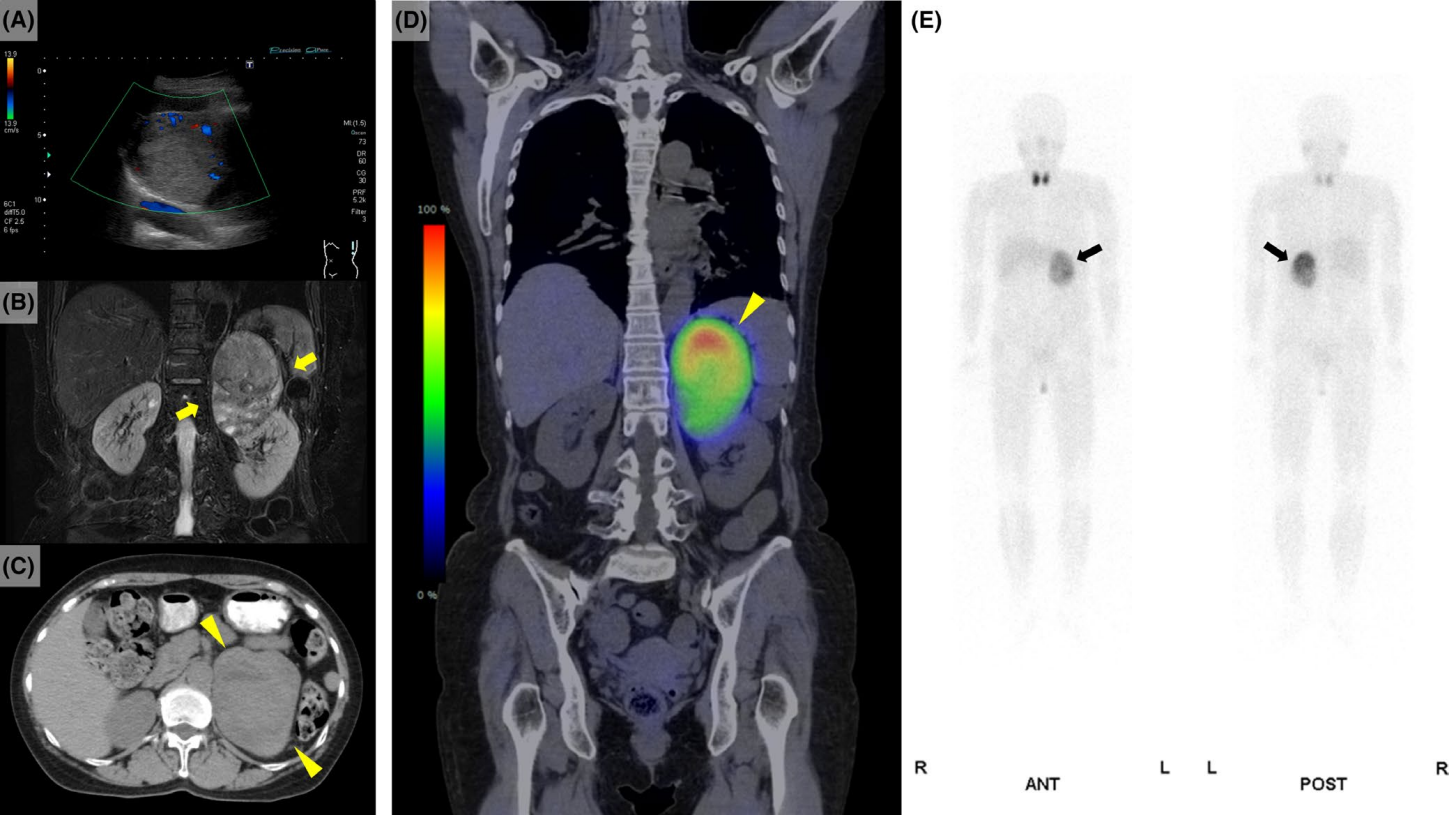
A, Abdominal ultrasonography showing a 11.8 × 7.8 cm massive hypovascular heterogeneous mass with cystic lesions in the left adrenal gland. B, Abdominal noncontrast computed tomography revealing a 10‐cm mass in the left adrenal gland (arrowheads). C, Abdominal fat‐suppression T2‐weighted magnetic resonance imaging showing a well‐circumscribed tumor suggestive of pheochromocytoma. No infiltration of the surrounding tissue is noted. D, E, ^123^I‐metaiodobenzylguanidine scintigraphy revealing intense uptake in the left adrenal tumor
